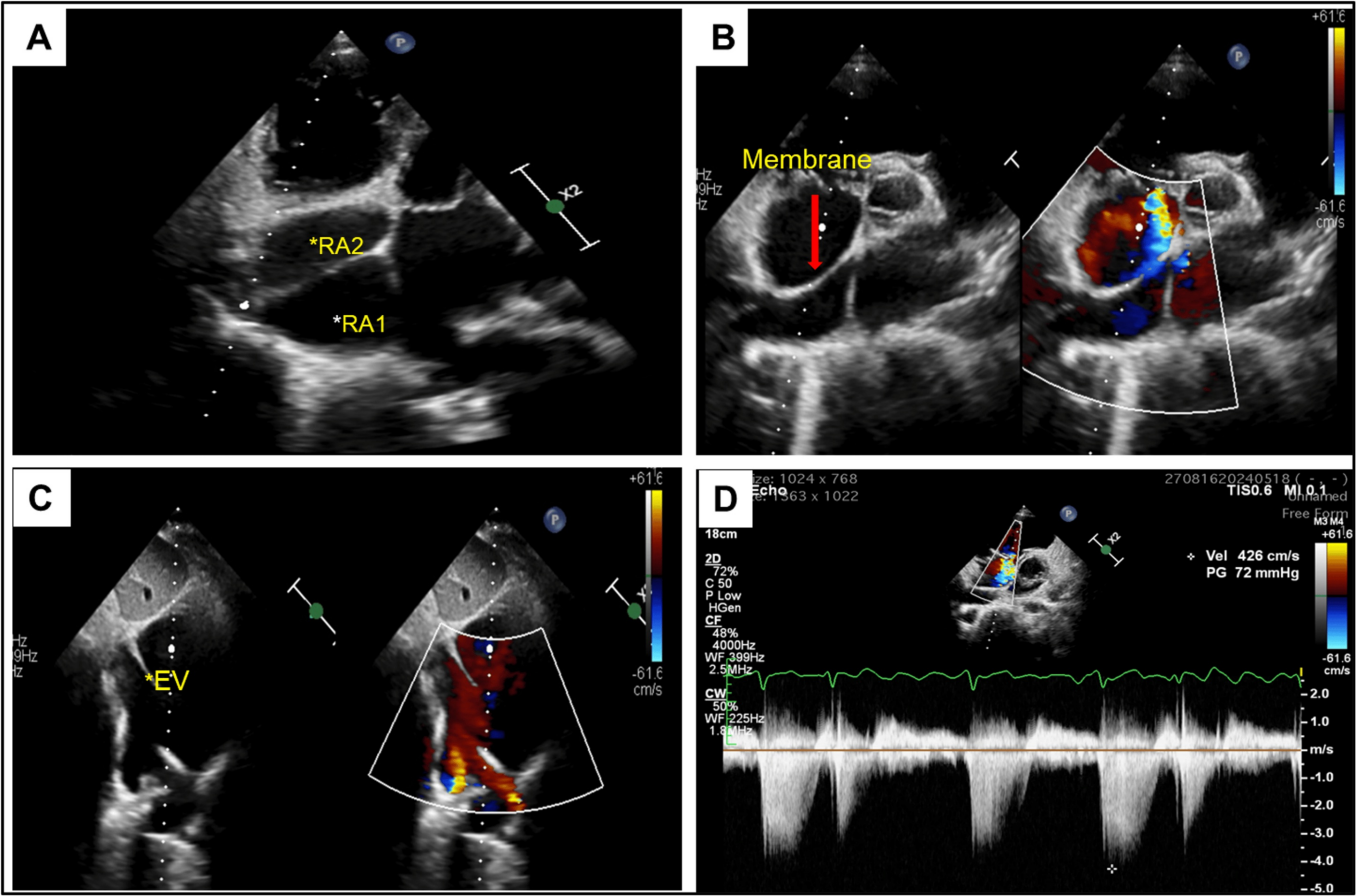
Transthoracic echocardiographic images. (A) Modified off-axis apical four-chamber view showing a membrane dividing the right atrium into two chambers right atrium 1 (RA1) and right atrium (RA2) giving triatrial appearance. (B) Parasternal short-axis view showing the interatrial septum and a tissue band arising posteriorly, attaching to the interatrial septum near the aortic end. (C) Subcostal bicaval view showing a prominent eustachian valve (marked as EV with an asterisk), atrial septal defect with left-to-right shunt. (D) Continuous wave Doppler across tricuspid valve showing severe tricuspid regurgitation and severe pulmonary arterial hypertension.
